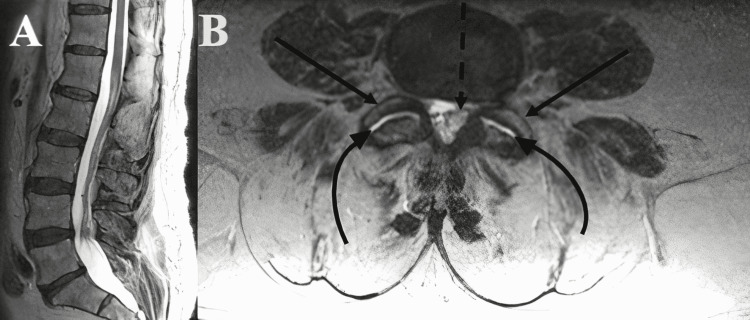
Preoperative MRI of the lumbar spine Sagittal image (A) and axial image (B) at L4/5 with bilateral facet arthropathy with facet effusion and left medial synovial cyst. MRI: magnetic resonance imaging; dashed arrow: left medial synovial cyst; curved arrow: bilateral facet effusion; straight arrow: bilateral facet arthropathy
